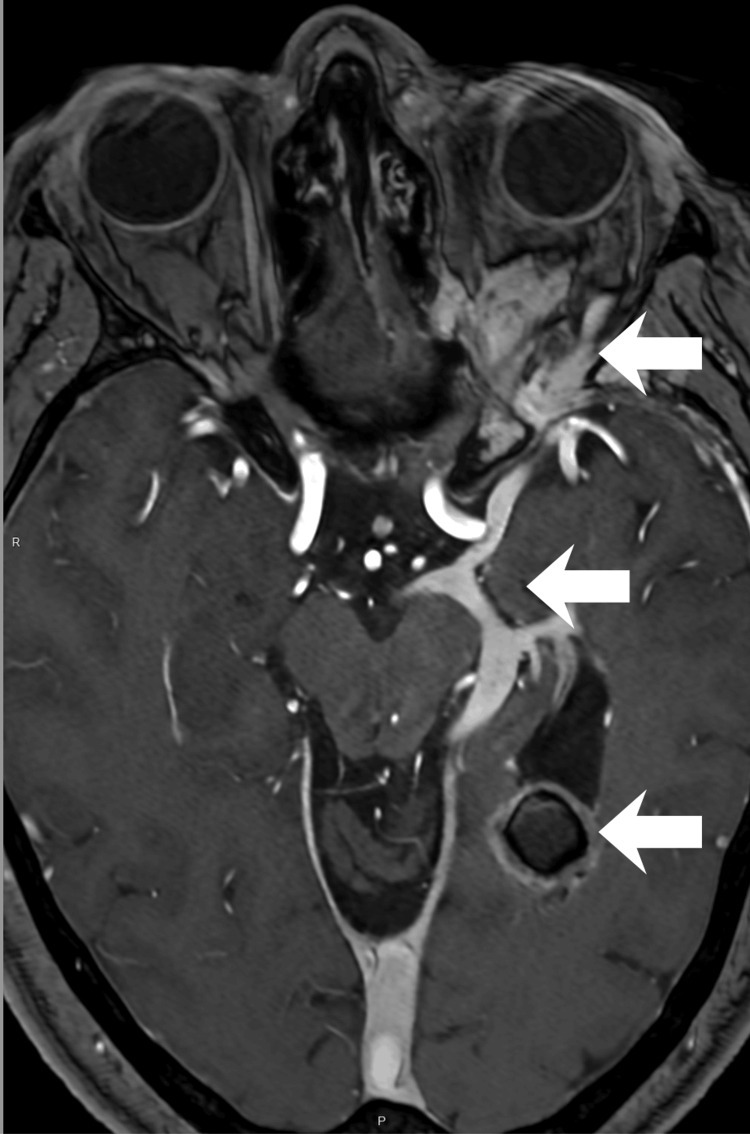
Orbital cavernous venous malformation and venous drainage MRI obtained six years after surgical resection of the primary orbital lesion showing a contrast-enhancing heterogeneous lesion in the posterior left orbit, associated with ectatic and tortuous vascular structures draining to the superficial medial cerebral veins through the superior orbital fissure, connecting to a dilated basal vein of Rosenthal and to the cavernous malformation in the left lateral ventricle. MRI: magnetic resonance imaging
